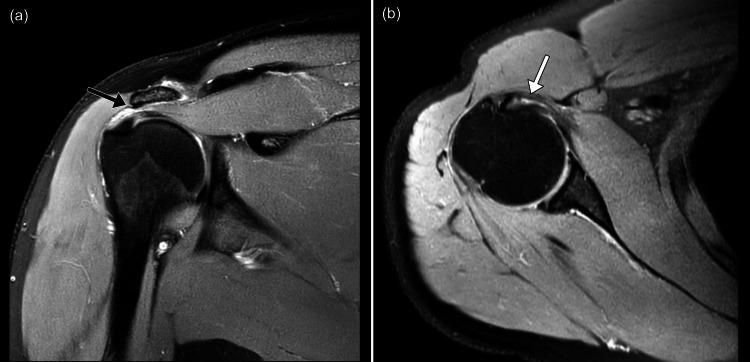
MRI of the right shoulder, proton density weighted images, (a) coronal view and (b) axial view. Black arrow: acromial spur impinging on supraspinatus tendon; white arrow: intrasubstance tendinosis of the subscapularis tendon.
